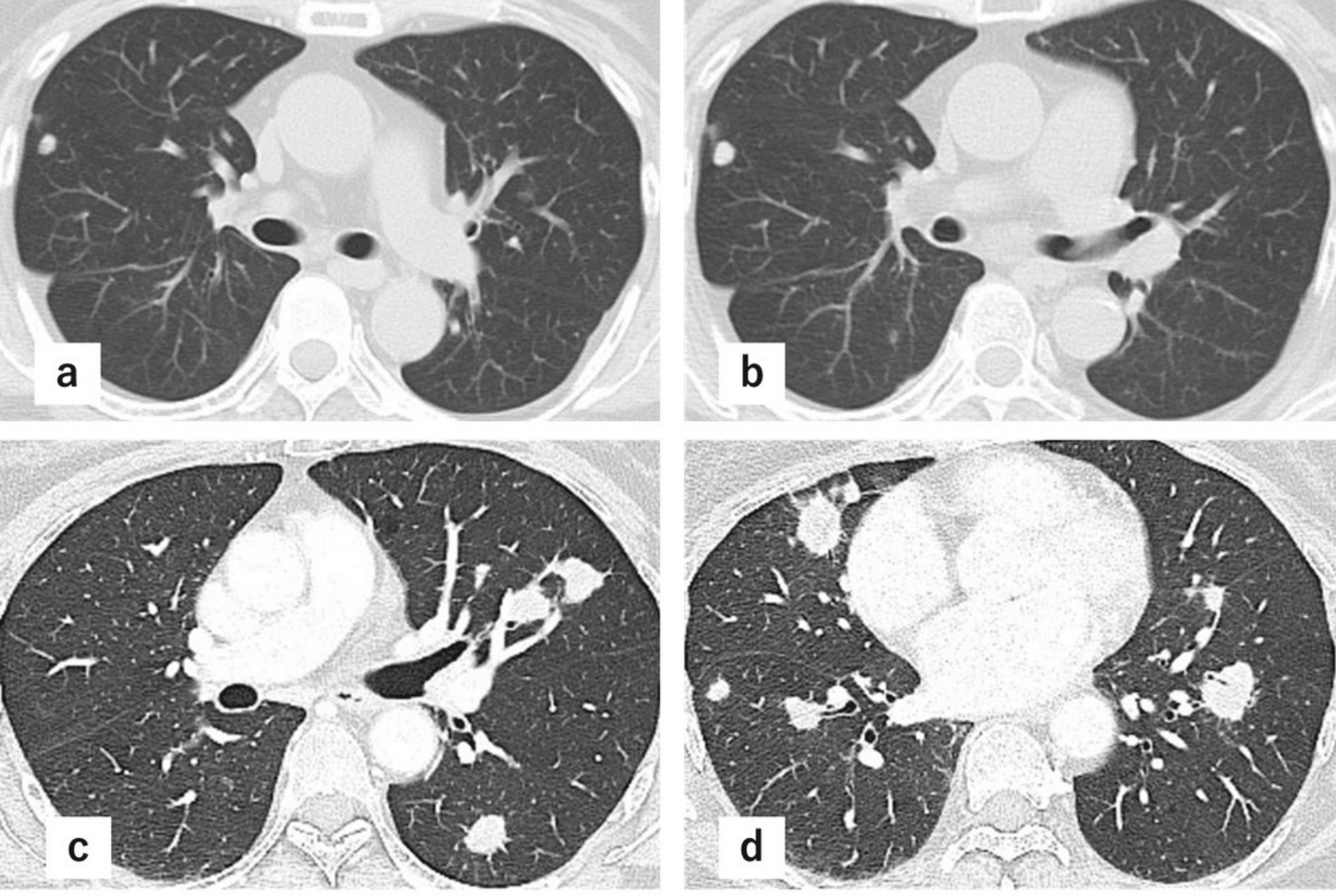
Preoperative computed tomography imaging. The computed tomography imaging findings are described as follows: Case 1 demonstrated gradually enlarging nodules from September 2020 (a) to September 2023 (b), and Case 2 demonstrated multiple bilateral pulmonary nodules measuring approximately 1-3 cm in diameter in February 2024 (c and d).
